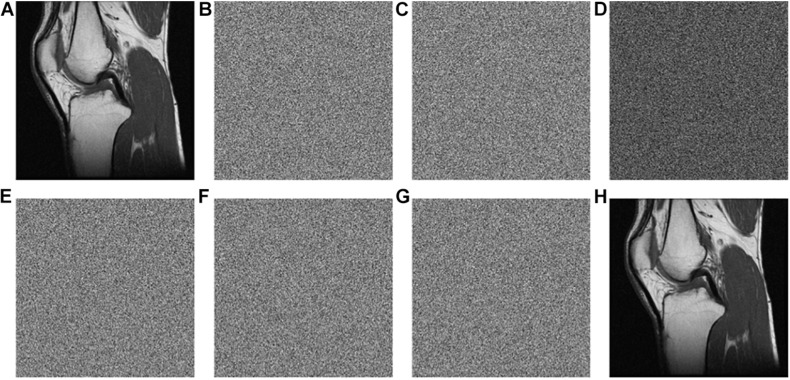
Test of key sensitivity. (A) The original image of “MRI-knee joint.” (B) The encrypted image with the initial key set. (C) The encrypted image with x0+t. (D) The differential image between (B,C). (E) The decrypted image with x0+t. (F) The decrypted image with ${\text{R}}_{\text{h}}^{\prime }+1$. (G) The decrypted image with the hash value + “1.” (H) The decrypted image with the correct key.
